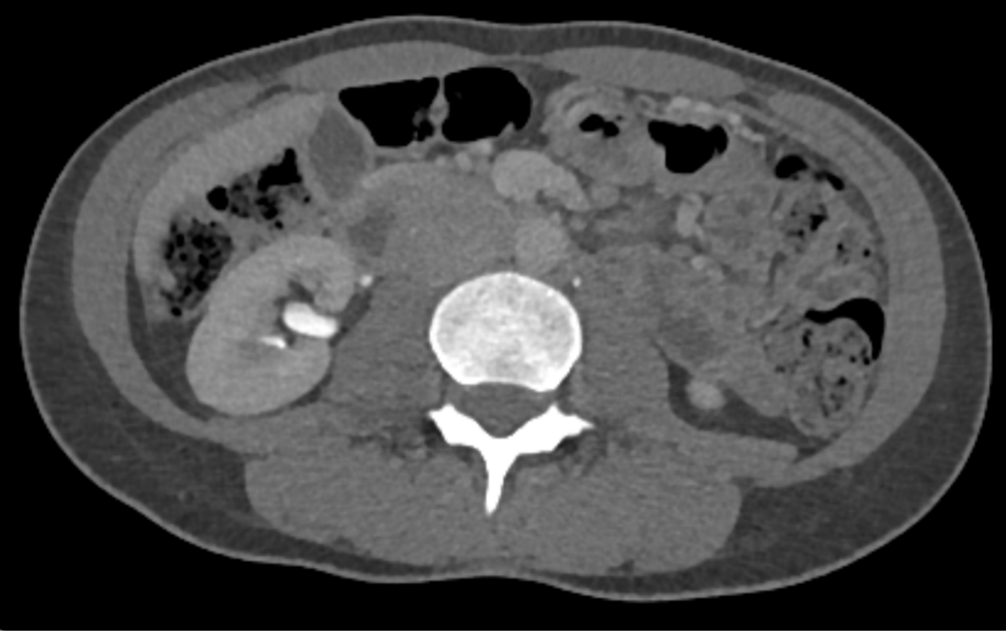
CT-IVU demonstrating resolution of the right renal pelvic haematoma with no
